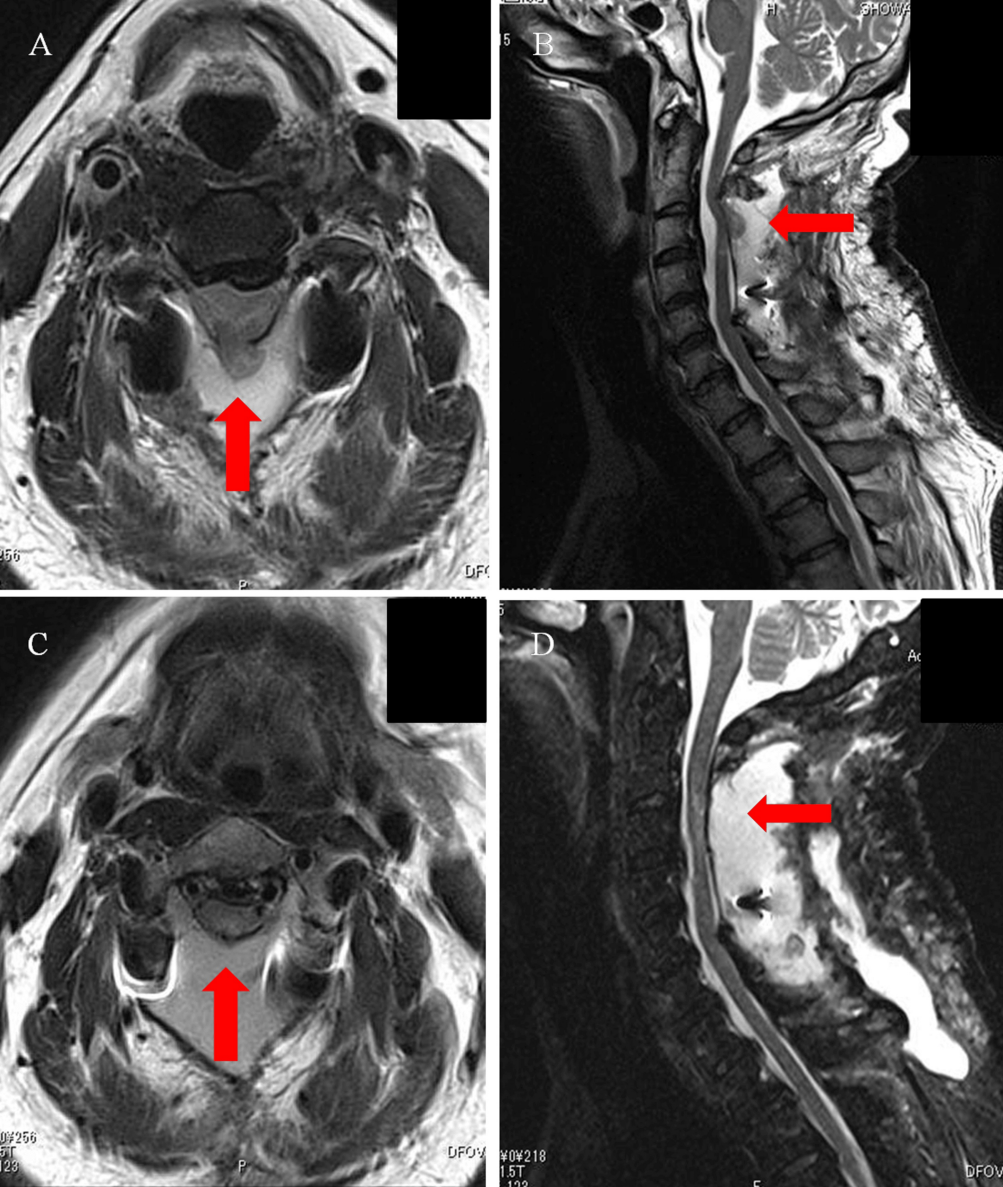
Postoperative MRI T2-weighted images of the cervical spine and postoperative MRI T2-weighted images of the spinal hernia return (A) Sagittal section and (B) horizontal section showing a deviation of the spinal cord (red arrows) from the dorsal interdural space at the C2/3 level. (C) Sagittal section and (D) horizontal section demonstrating retraction of the spinal cord hernias shown in (A) and (B) (red arrows).
